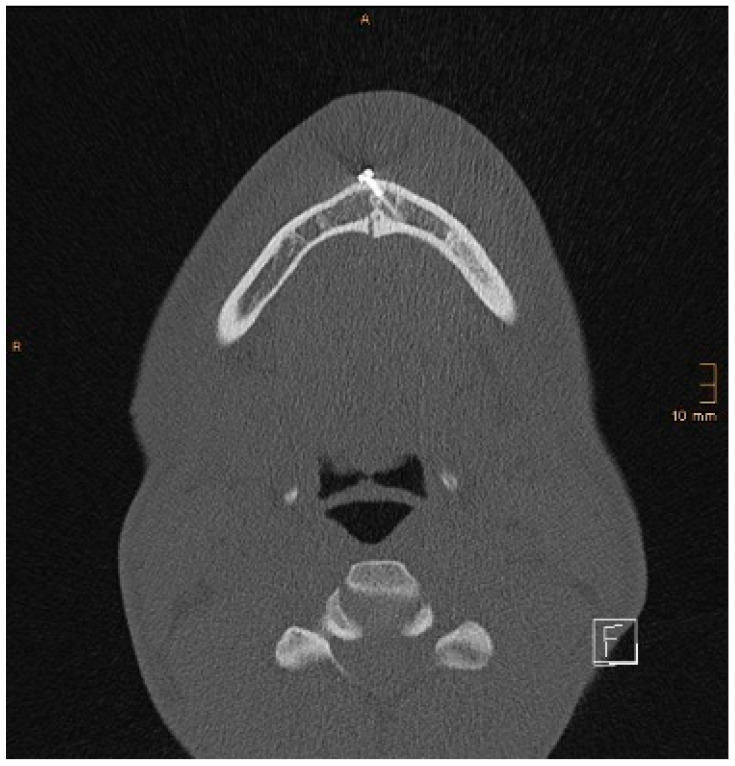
Axial CT scan showing the same patient as in Figure 3 after replacing the miniplates with a lag screw and considerable closure of the lingual gap. However, a small gap in the lingual cortical bone remained, and possibly determined a widening of the mandible.
